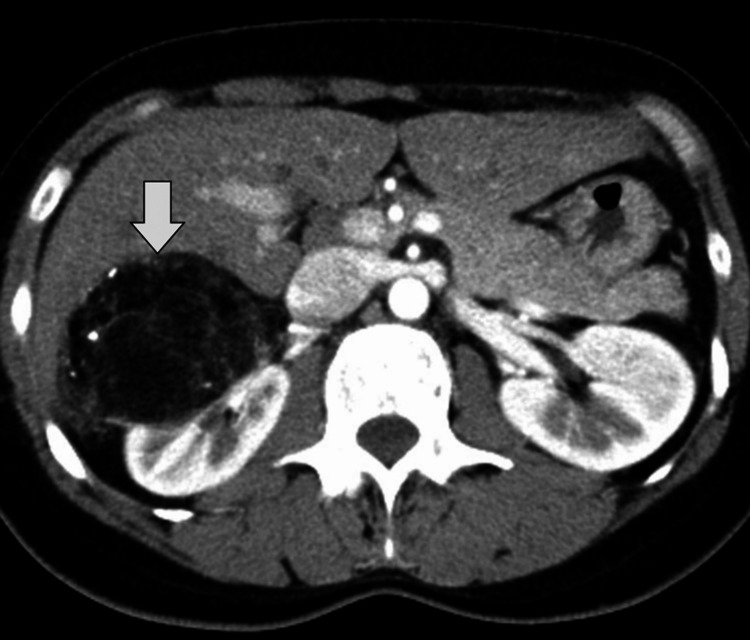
Post-embolization follow-up imaging Axial contrast-enhanced CT image obtained after embolization demonstrates a marked interval reduction in the size of the right renal angiomyolipoma (arrow), indicating successful treatment response.
